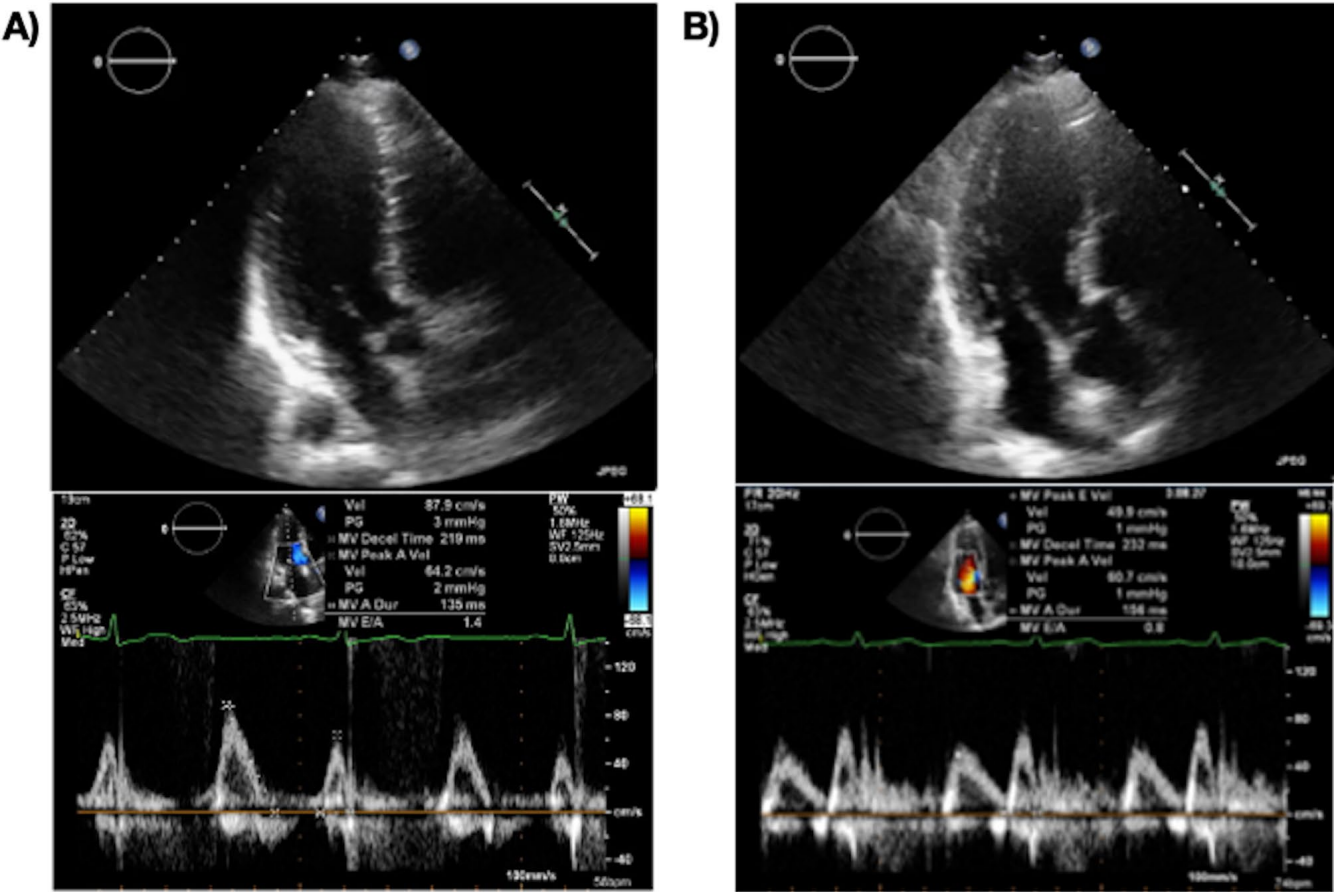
Representative early advanced-age cases with (A) normal cardiac size and normal inflow pattern, and, (B) abnormal relaxation pattern. Upper panel: Three-camber view. Lower panel: Transmitral inflow by pulse Doppler method.
